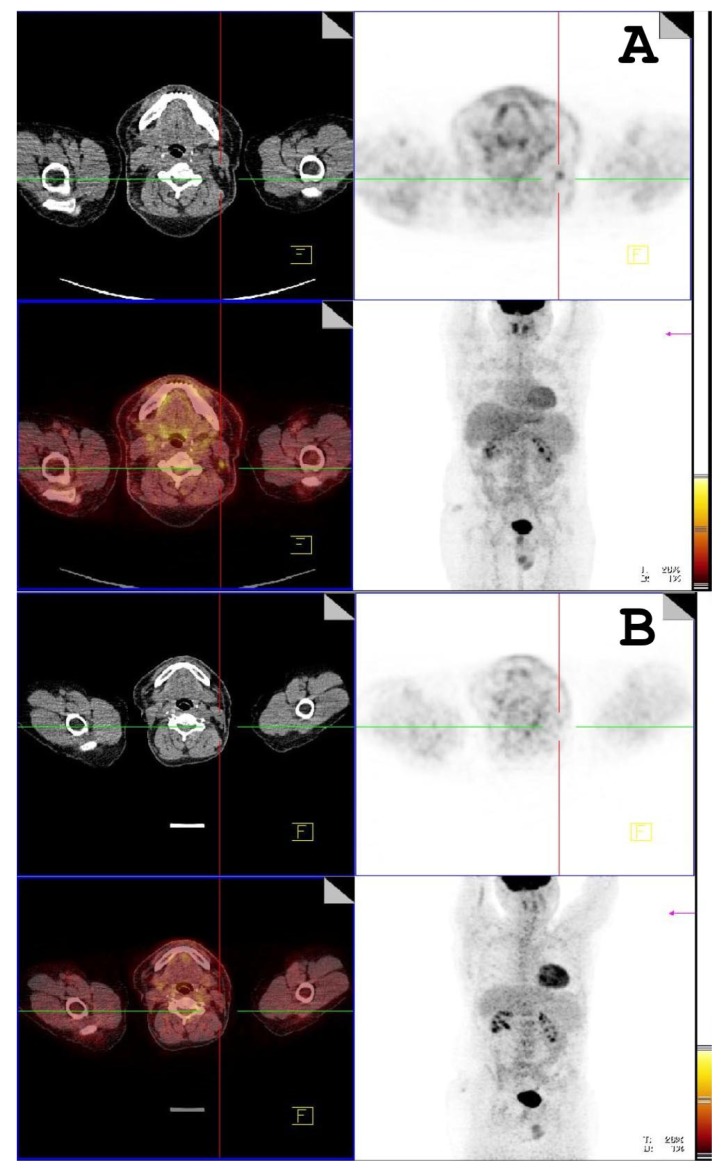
(A) First FDG PET/CT scan, active disease (sarcoidosis) present in the left cervical lymph node (B) Follow-up FDG PET/CT scan, total remission of disease after therapy.
